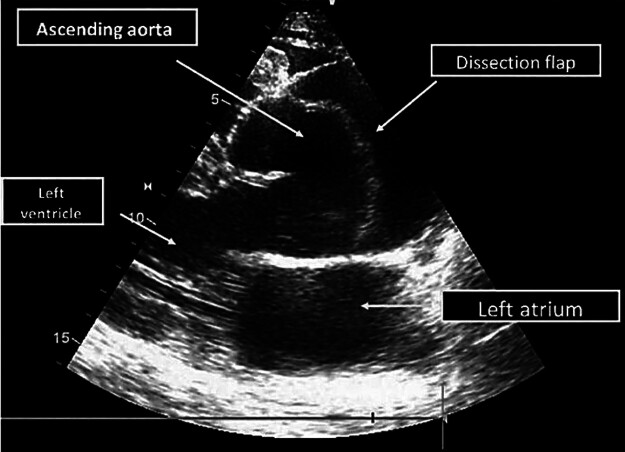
Two dimensional Echocardiography depicting dissection flap in ascending aorta.
